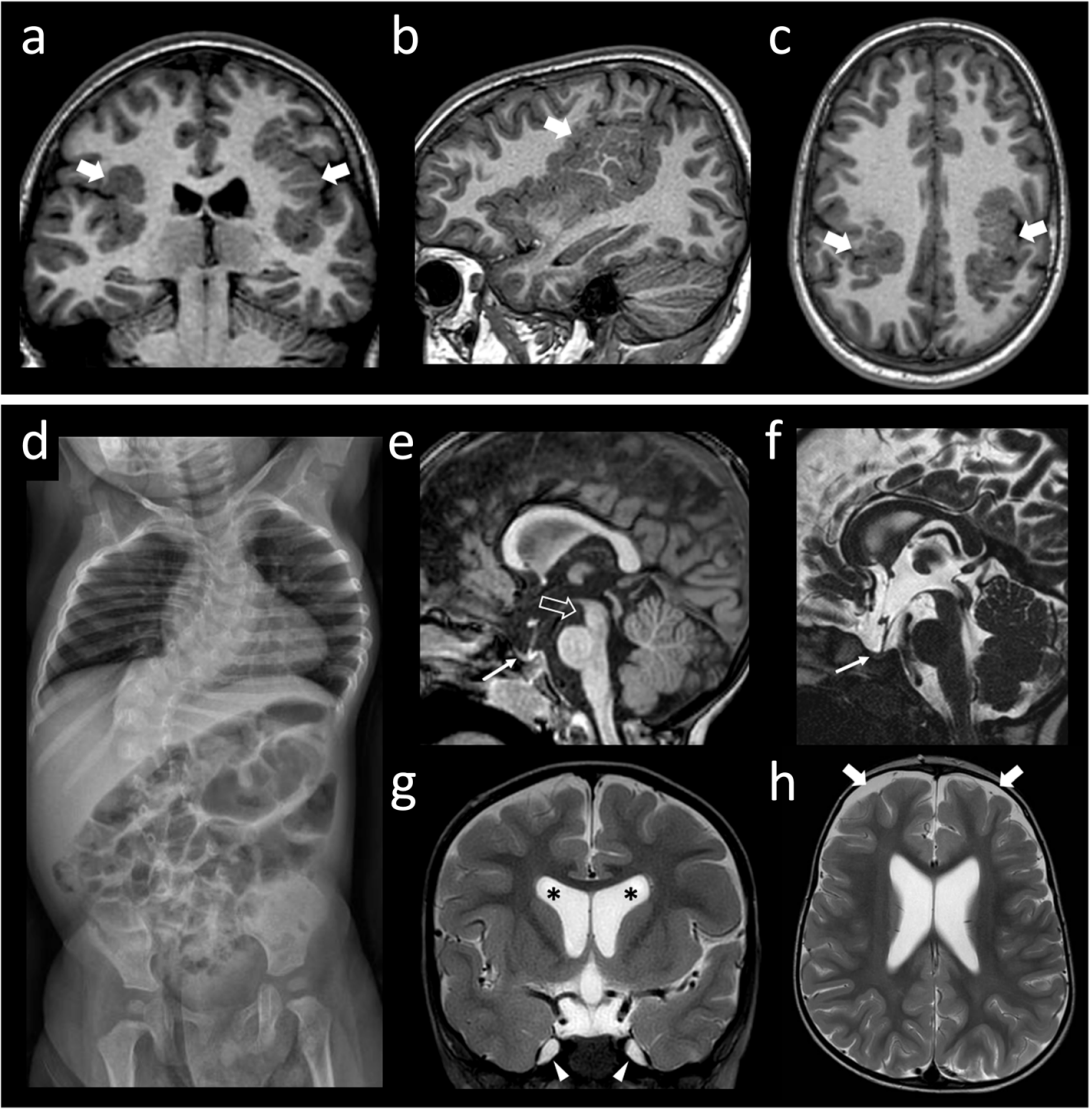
Radiological features. a-c) Brain MRI of Patient 1 performed at 13 years of age. a) Coronal, b) sagittal, and c) axial 3D T1-weighted reformatted images reveal bilateral polymicrogyria involving the insula and perisylvian regions, slightly more extended on the left side (arrows). d-h) X-ray and brain MRI of Patient 2 performed at 2 years of age. D) Long-spine plain film in the AP projection shows the severe dorsolumbar scoliosis. e) Sagittal T1-weighted and f) DRIVE (DRIVen Equilibrium) T2-weighted images demonstrate the hypoplasia of the adenohypophysis (arrows) associated with a minor dysmorphism of the brainstem characterized by a shorter midbrain (empty arrow). g) Coronal and h) axial T2-weighted images reveal thinning of the periventricular white matter with enlargement of the lateral ventricles (asterisks) and frontal subarachnoid spaces (thick arrows). Note the enlargement of the Meckel's caves (arrowheads)
